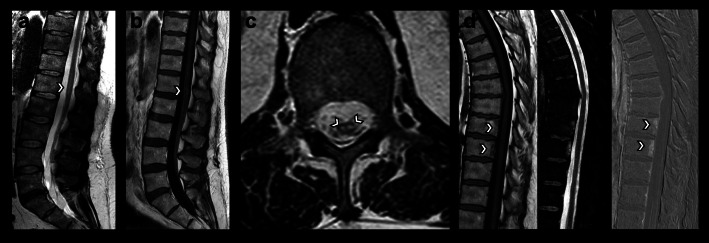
Contrast-enhanced follow-up MRI performed 4 weeks after symptom onset. a Sagittal T2-weighted image showing vertical linear foci of high T2-weighted signal located anteriorly within the lower spinal cord (arrow). b Sagittal T1-weighted image after contrast injection demonstrating newly occurred intramedullary contrast enhancement (arrow) and persisting enhancement of the conus medullaris and descending nerve roots. c Axial T2-weighted image revealing bilateral circular foci of high T2-weighted signal in the anterior portion of the spinal cord at L1 level (arrows), also referred to as owl-eyes sign, suggestive of a mature infarction. d Sagittal T1-weighted image without contrast mean (left), sagittal T2-weighted fat-suppressed image (middle) and sagittal T1-weighted subtraction image after gadolinium-based contrast mean injection subtracted by the native T1-weighted image showing focal oedema in the posterior portion of the Th9 and Th10 vertebral bodies with associated contrast uptake, suggestive of vertebral body infarction
